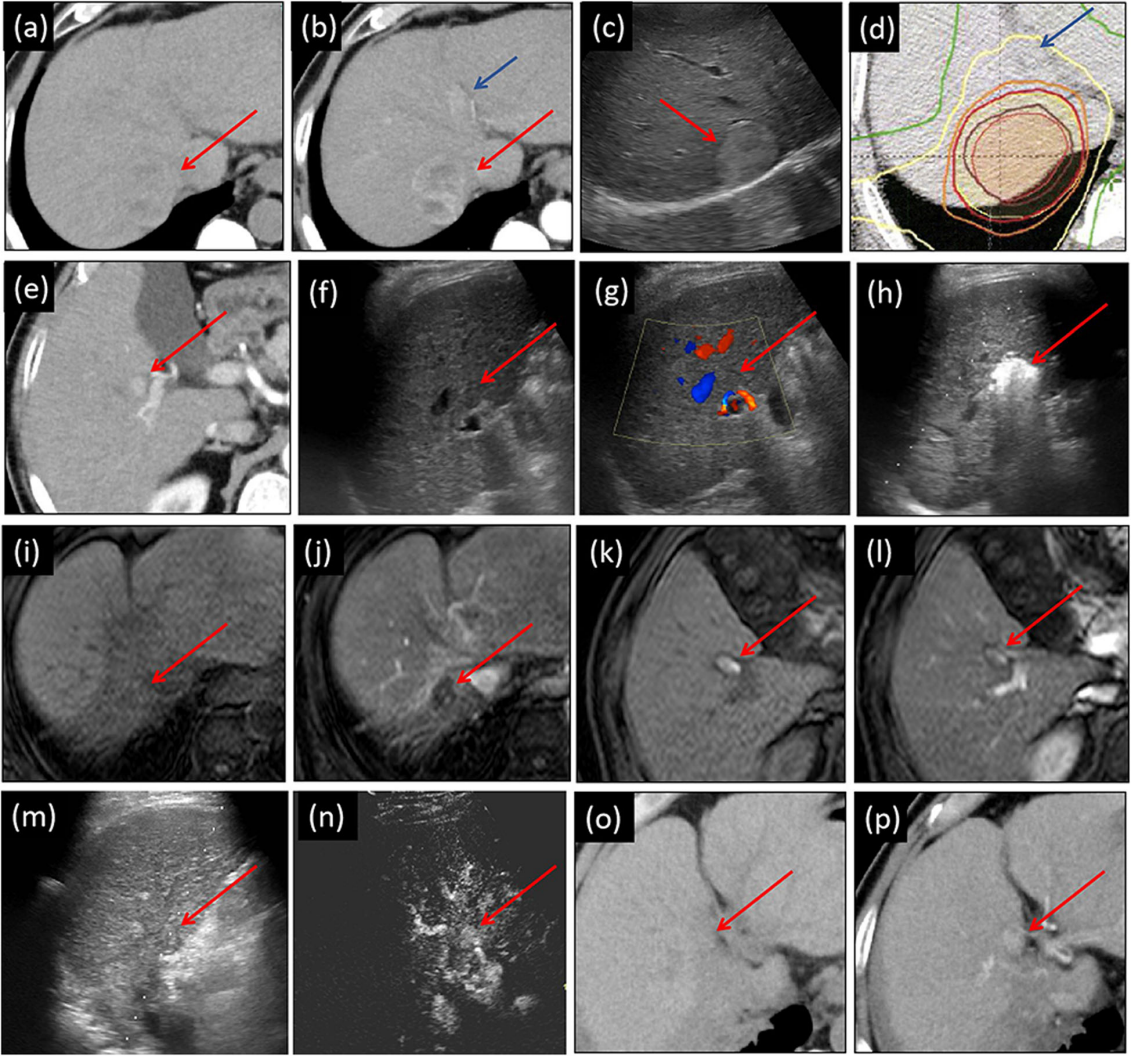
A case of complete tumor response and intrahepatic distal recurrence. (a,b) A 40 mm lesion in segment (S)7 was planned for SBRT, showing unenhanced CT and arterial phase (AP) of contrast-enhanced CT images, respectively. The lesion was partial hyperenhanced. (c) Grayscale ultrasound (US) image shows the lesion in S7 located adjacent to the diaphragm and hepatic vein. It was estimated that ablation would be risky because of its location. The lesion was well-defined and with a nodule-in-nodule appearance. (d) A dose distribution picture of SBRT treatment plan was generated. A total dose of 40 Gy (red isodose line) was delivered in 5 fractions. The central part received 55 Gy radiation. (e) An 11 mm lesion located in S5 was detected in the AP of the contrast-enhanced CT image. (f,g) Grayscale US and color Doppler flow image of the lesion. (h) Grayscale US image in the process of RFA. (i,j) Compared with unenhanced T1-weighted MR image (i), at the one-year follow-up, the SBRT-treated area considerably decreased and changed into totally hypoenhancement (j). (k,l) At the one-year follow-up, the RFA-treated area changed into hyperenhanced scars in both the unenhanced T1-weighted image (k) and the AP of Gadolinium-Ethoxybenzyl-Diethylenetriamine Pentaacetic Acid MRI (EOB-MRI) (l). (m,n) After 44.8 months’ follow-up, a new 10 mm lesion was detected in S4. In grayscale US (m), it appeared as slightly hyperechoic and poorly defined. In the AP of contrast-enhanced US (n), the lesion displayed hypervascularity. In the unenhanced CT(o) taken as a reference, the AP of the contrast-enhanced CT image (p) showed hyperenhancement. Red arrows in (a–c) and (e–p) indicate the location of the target lesion or post-treated area. Dark blue arrows in (b) and (d) show the approximate position of the S7 lesion. This case corresponds to the No. 1 patient shown in the tables
